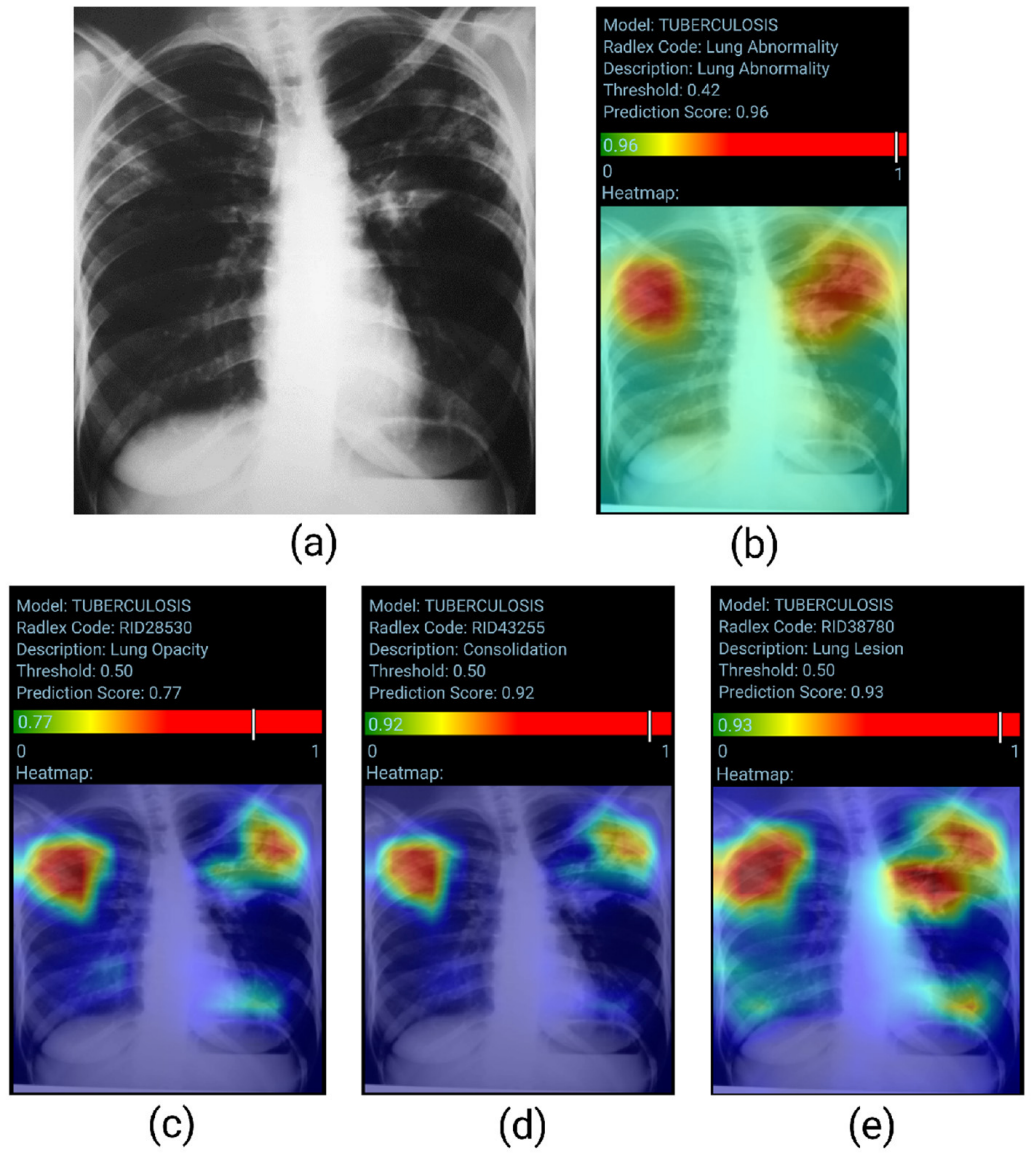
True positive sample for the Lung Abnormality and the Radiological Findings Models. (A) Shows original chest-x-ray, (B) shows the LAM result, and (C–E) show the result for three findings for RFM.
